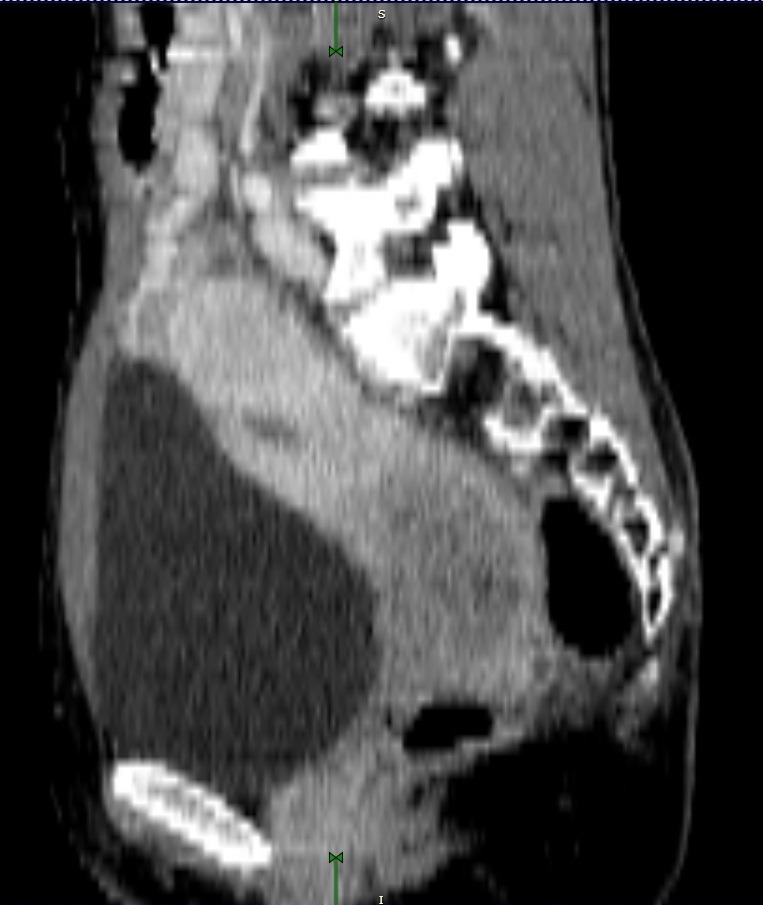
CT sagittal view of the patient’s pelvis before EBRT (anteverted position of the uterus)
